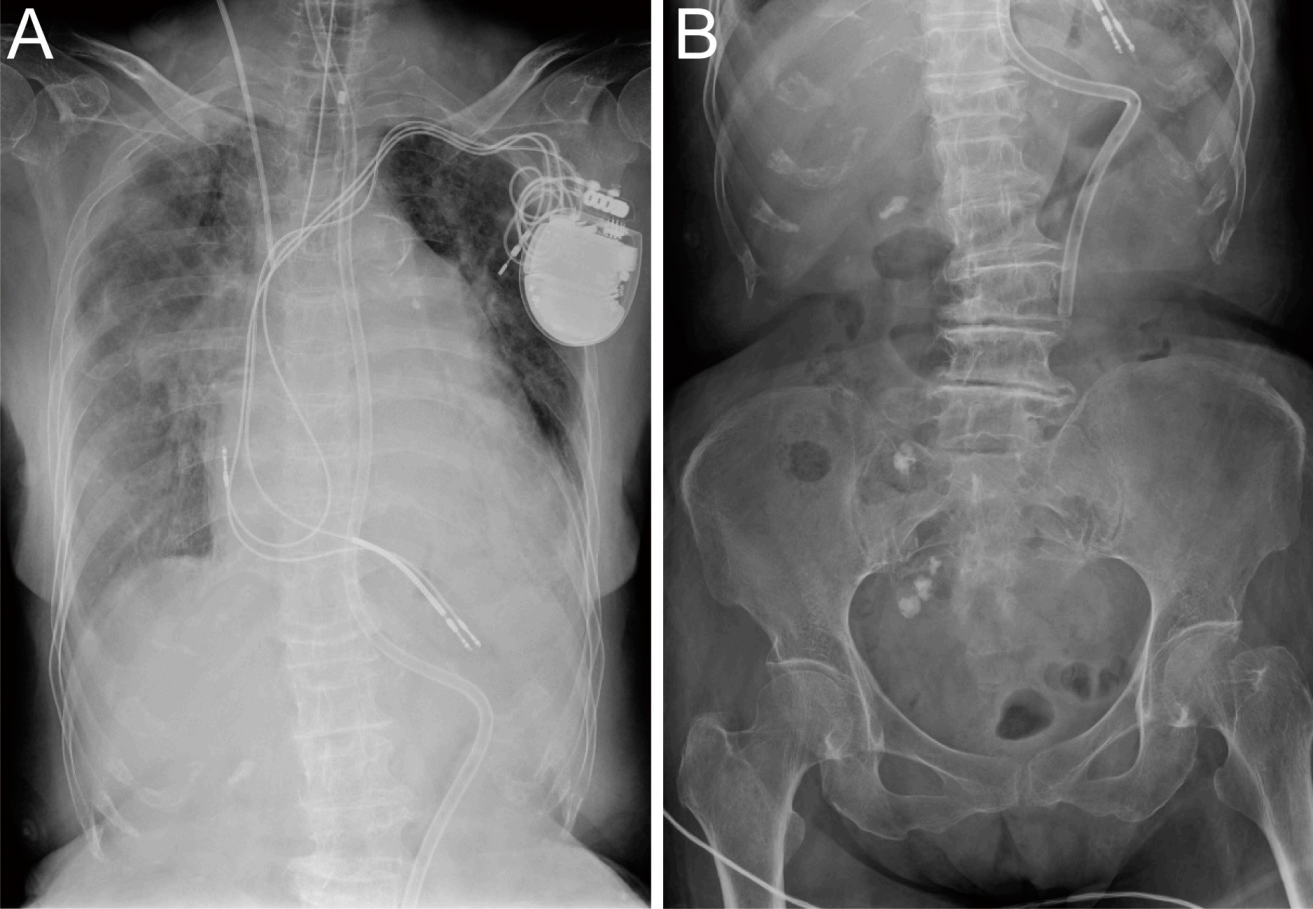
Radiography at the admission. A: Chest radiography revealed lung consolidation. Note that the patient is endotracheally intubated and a nasogastric tube is inserted. B: Abdominal radiography. The nasogastric tube tip was beyond the diaphragm, and the tube was fixed at the nasal cavity at 65 cm. Note: the height of the patient is 153 cm.
